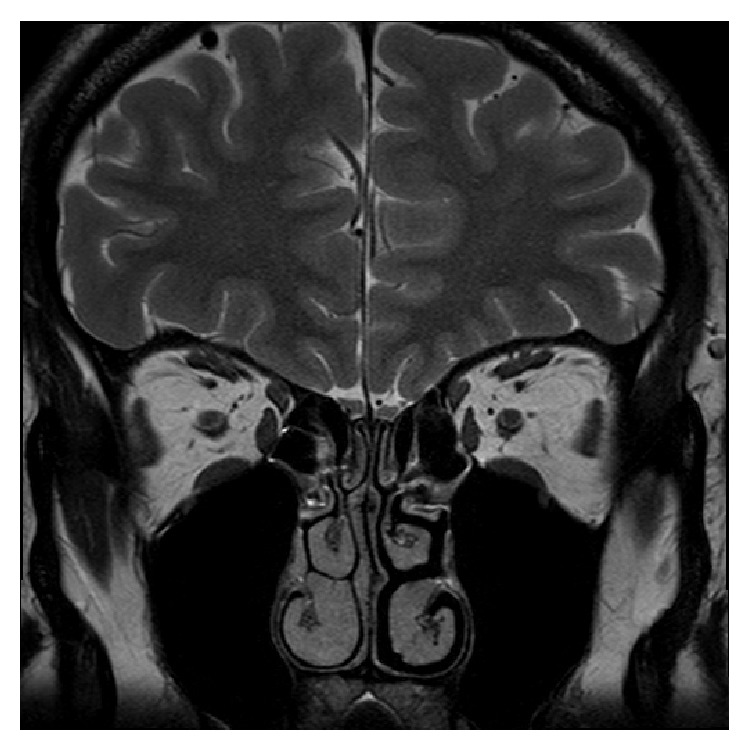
Magnetic resonance imaging showed mild sinusitis with normal brain structures.
